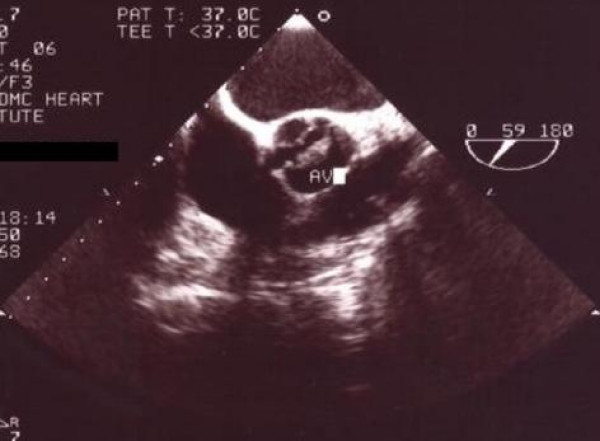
Long axis transthoracic echocardiography of patient showing echogenic mass on ventricular side of aortic valve attached to the right coronary leaflet (vegetation).
